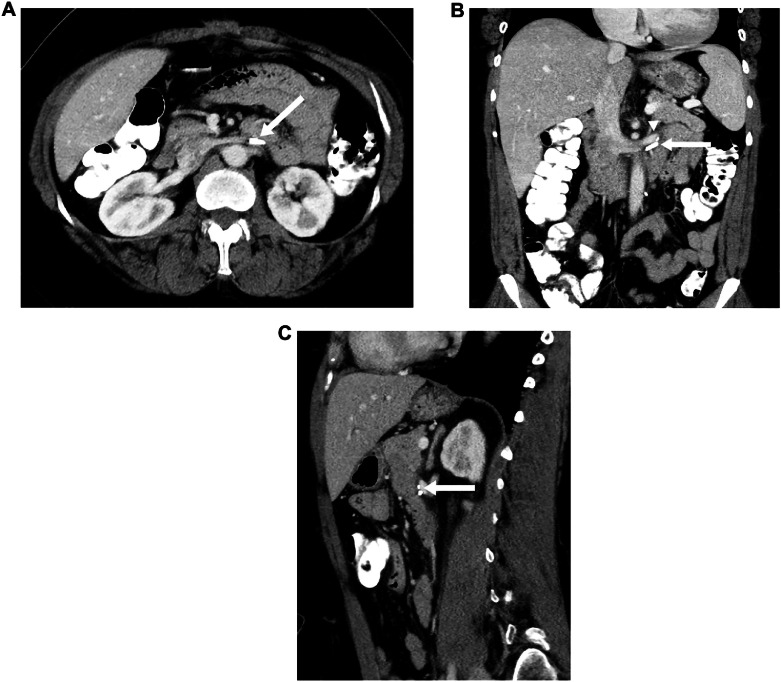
(A) Axial, (B) coronal, and (C) sagittal CT images of the abdomen with IV contrast 1 year after surgery demonstrate resection of the retroperitoneal mass. There is no evidence of residual or recurrent tumor. Surgical clips (arrows) are seen at the resection site, including two surgical clips along the left renal vein (arrowhead).
